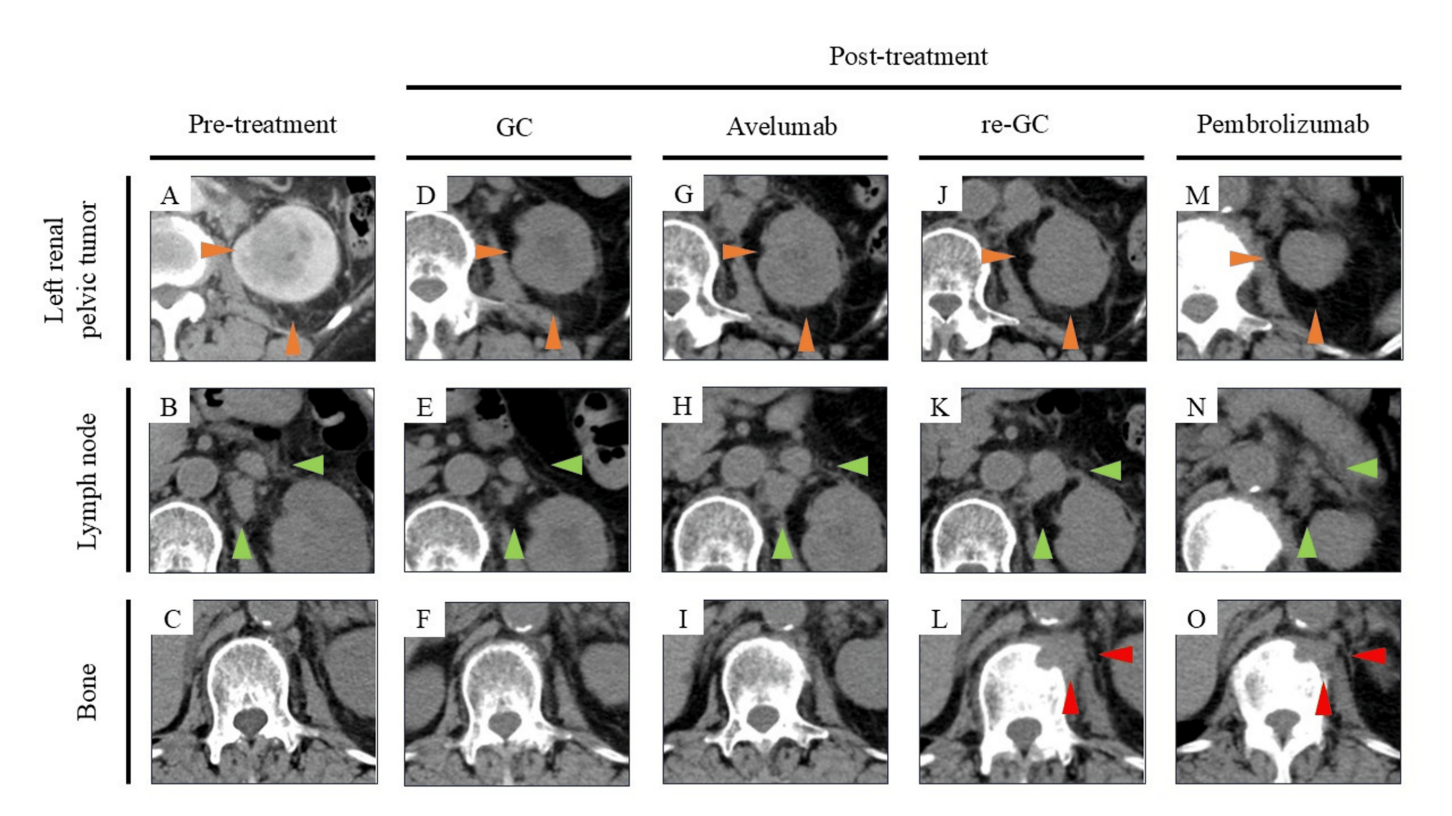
CT images of the left renal pelvic tumor, lymph node, and bone for every treatment Orange arrowheads show a left renal pelvic tumor. Green arrowheads show lymph nodes. Red arrowheads show bone metastasis. GC: gemcitabine and cisplatin combined chemotherapy, re-GC: rechallenged GC chemotherapy; CT: computed tomography
